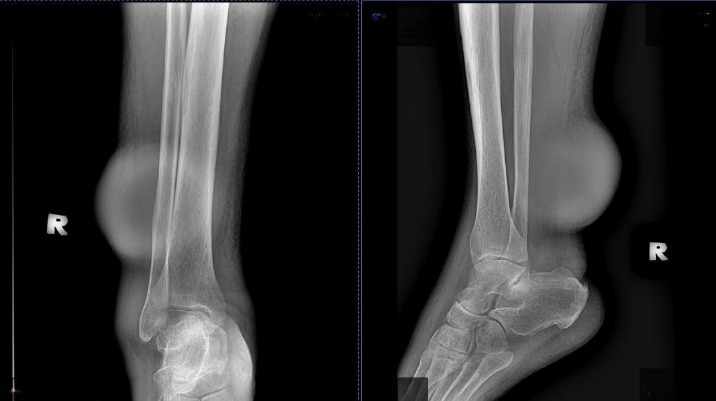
Radiographic view of the right lower limb (anteroposterior and lateral views).
